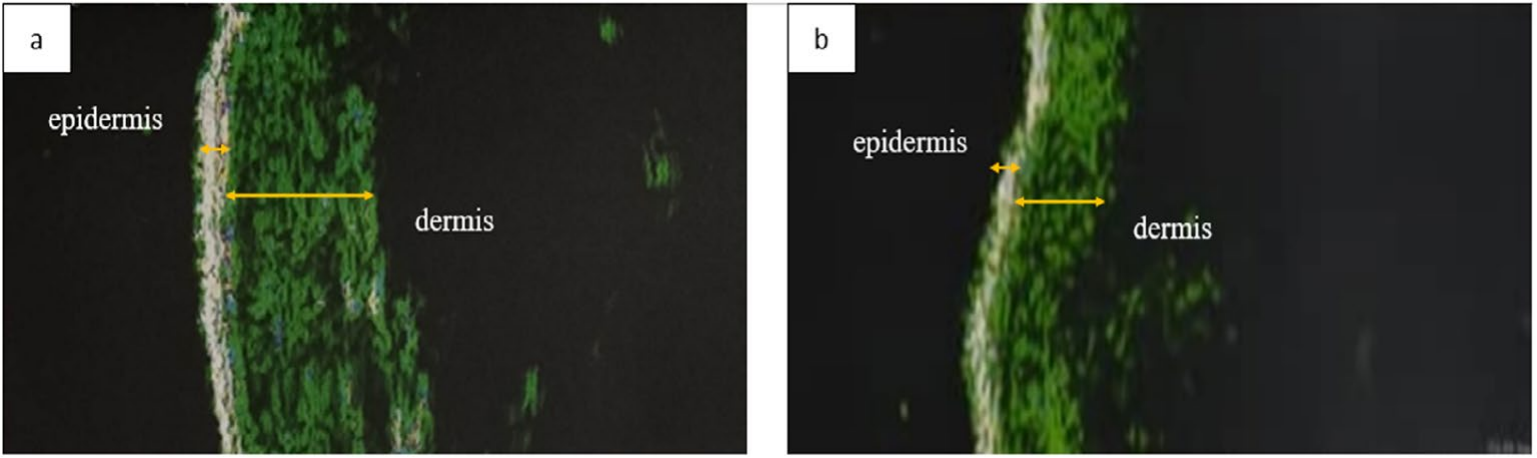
Ultrasound imaging of cutaneous lesions (a) before treatment (b) after treatment.
